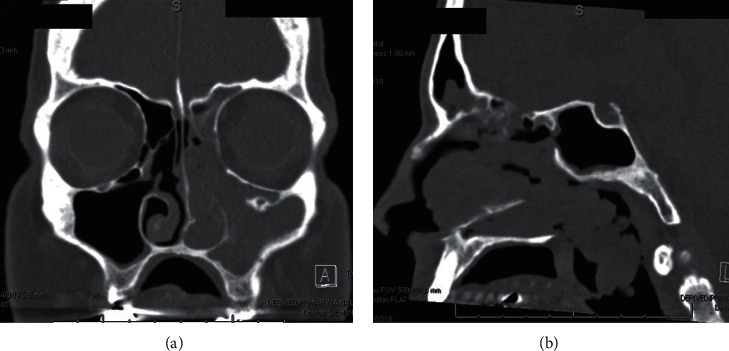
Coronal CT of sinonasal mass with complete opacification of left paranasal sinuses (a) and sagittal CT of mass extending from the nasal cavity down to oropharynx in contact with the soft palate (b).
